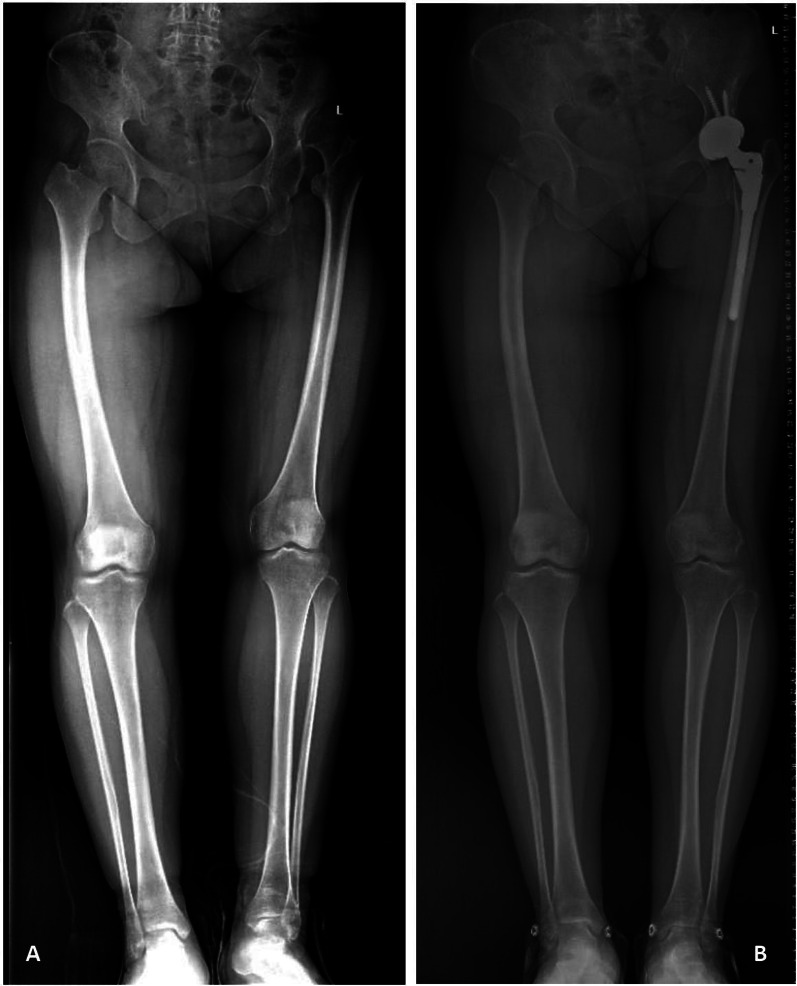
Non-valgus alignment of the knee in a 50-year-old Crowe type IV patient after total hip arthroplasty. A Preoperative radiograph. B Postoperative radiograph
